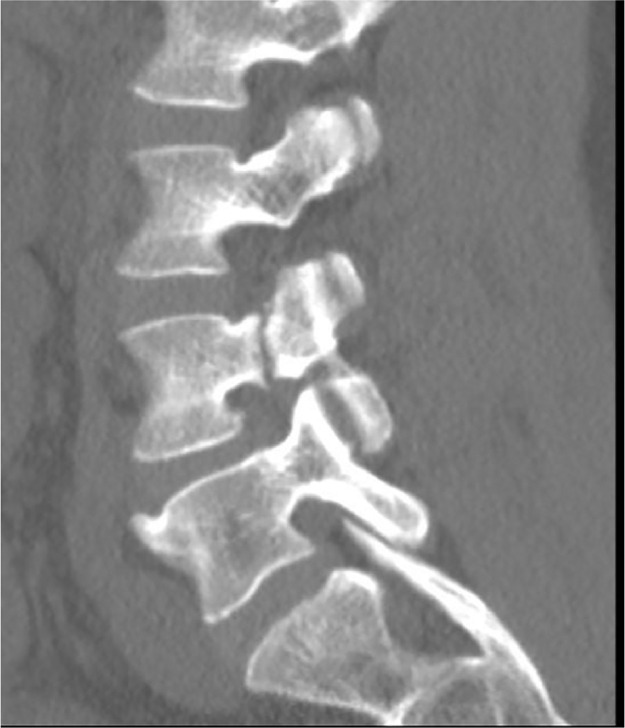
Sagittal CT image showing cranio-caudal extent of the fracture.
